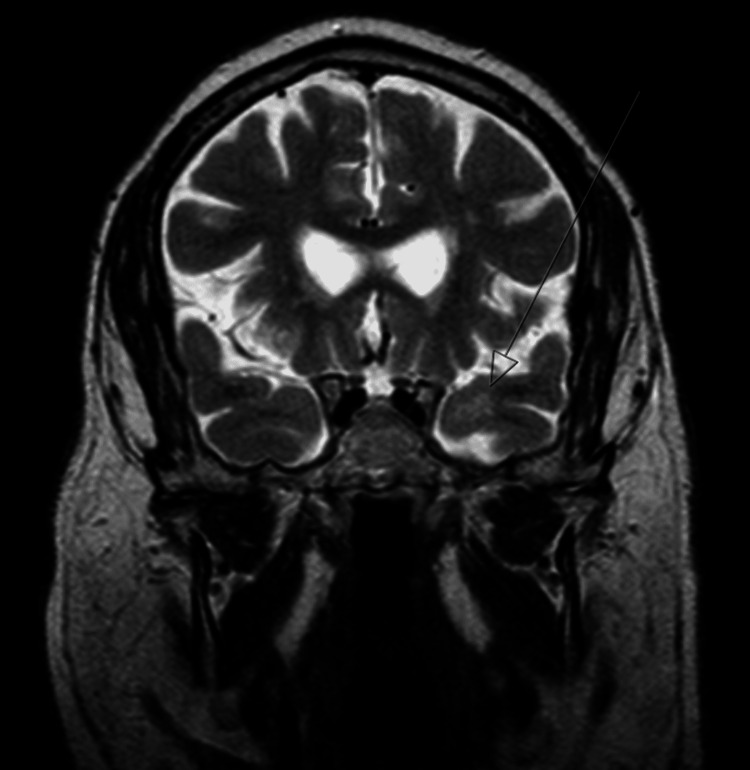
Brain MRI coronal T2 showing hyperintensity in the left anterior temporal lobe
